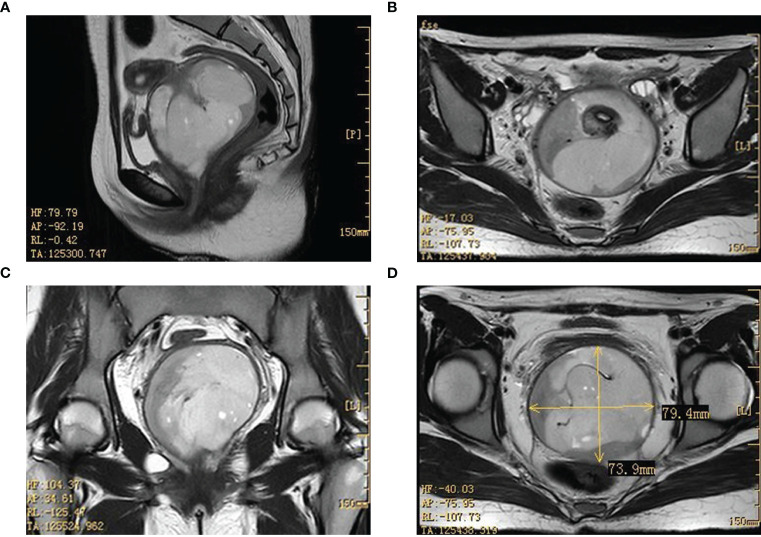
Imaging results in the pelvic lesion tissue. The results of the MRI showed a mixed signal mass in the external cervical orifice and vaginal fornix (size about 7.4×7.9×8.5cm). (A, C: sagittal position; B, D: horizontal position). MRI, magnetic resonance imaging.
